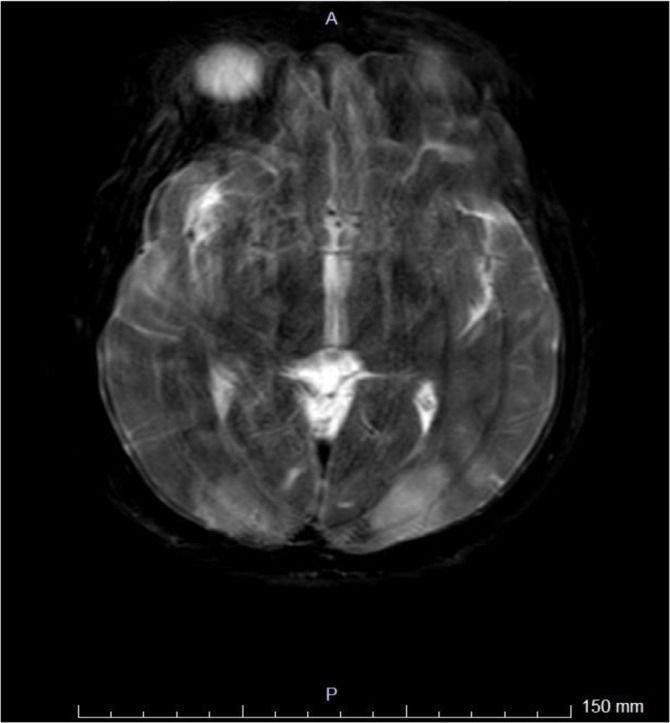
T2 weighted image of brain MRI 39-year-old man with systemic lupus erythematosus, presenting with headache and seizures after cytotoxic treatment and hypertension. Hyperintense lesions, posterior subcortical right side predominant vasogenic white matter edema is seen indicating PRES.
